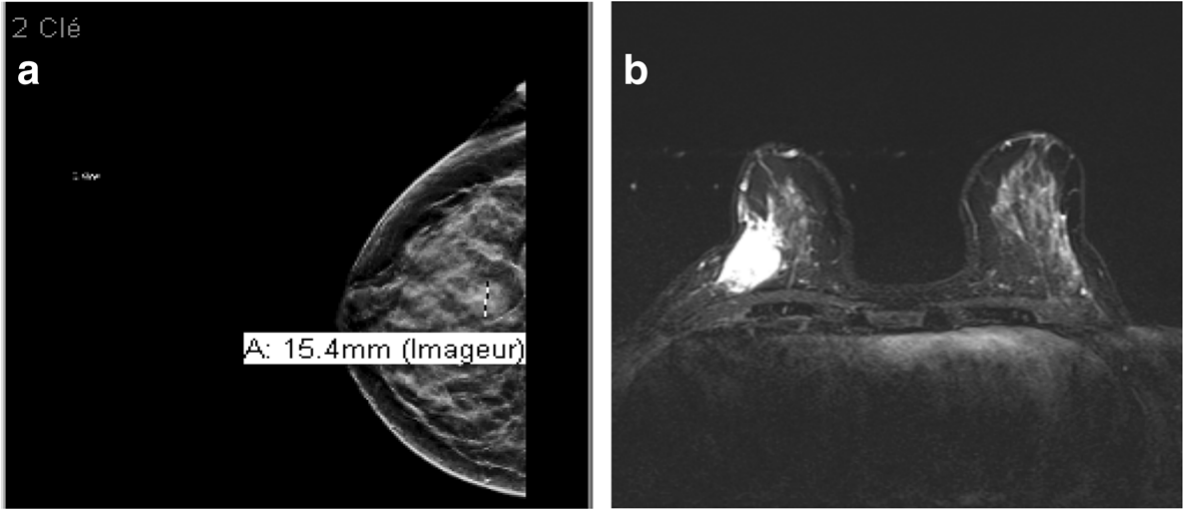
a Initial mammography. Well-delimited mass in the lower external quadrant of the right breast. b Initial magnetic resonance imaging. Mass in the lower external quadrant of 2.8 × 3.0 × 3.0 cm. From the subcutaneous plane to the pectoral plane with no infiltration of the pectoral muscle. Central necrosis with peripheral contrast enhancement
